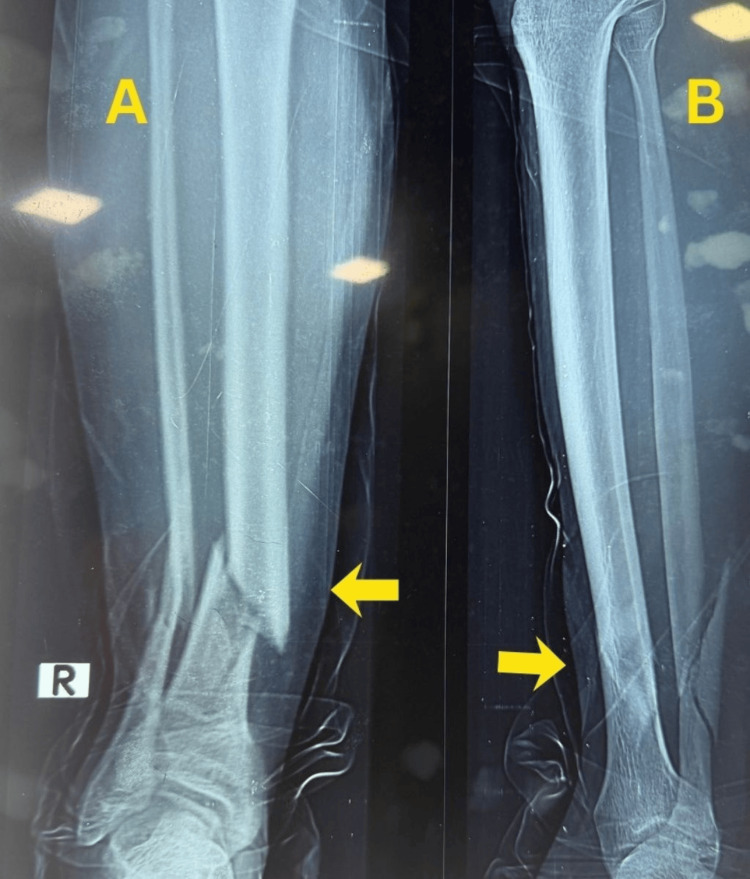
Preoperative radiographs of Case 1. (A) Anteroposterior view showing an extra-articular distal tibial fracture with an associated fibular fracture. (B) Lateral view demonstrating fracture configuration, displacement, and distal tibial involvement.
